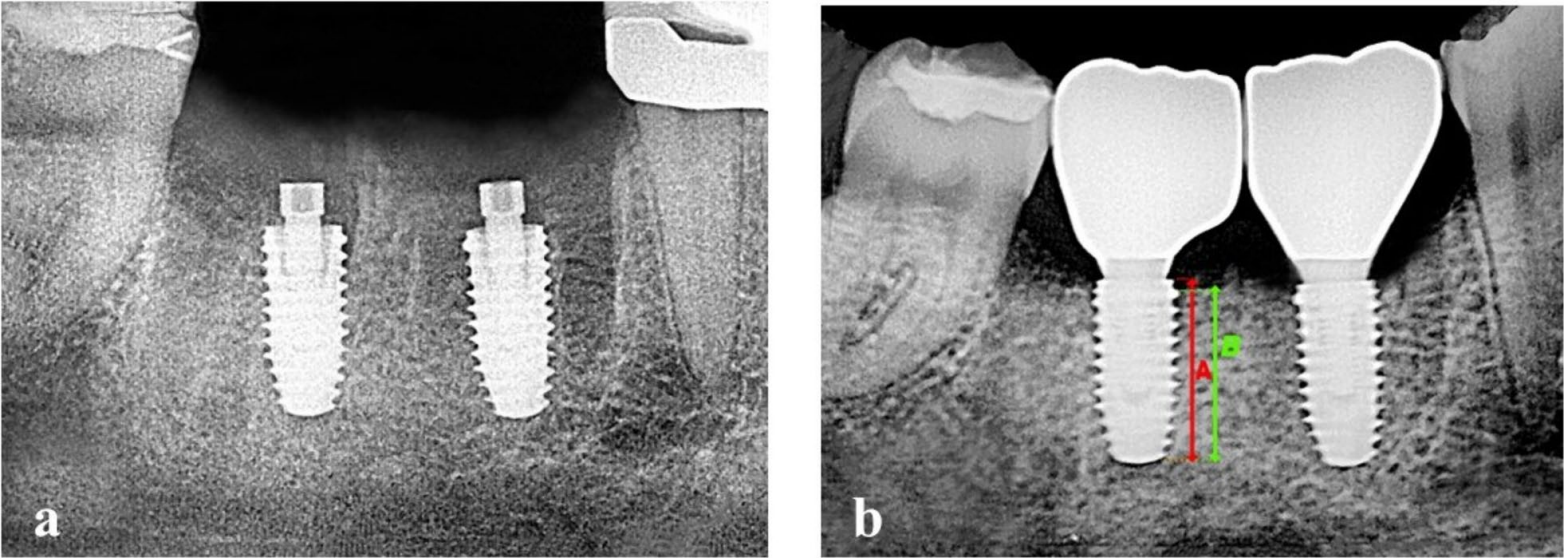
a, b Periapical X-ray using long cone parallel technique and a phosphor imaging plate instantly after the surgical procedure (T0) and at 12 months follow-up to measure marginal bone loss (MBL)
